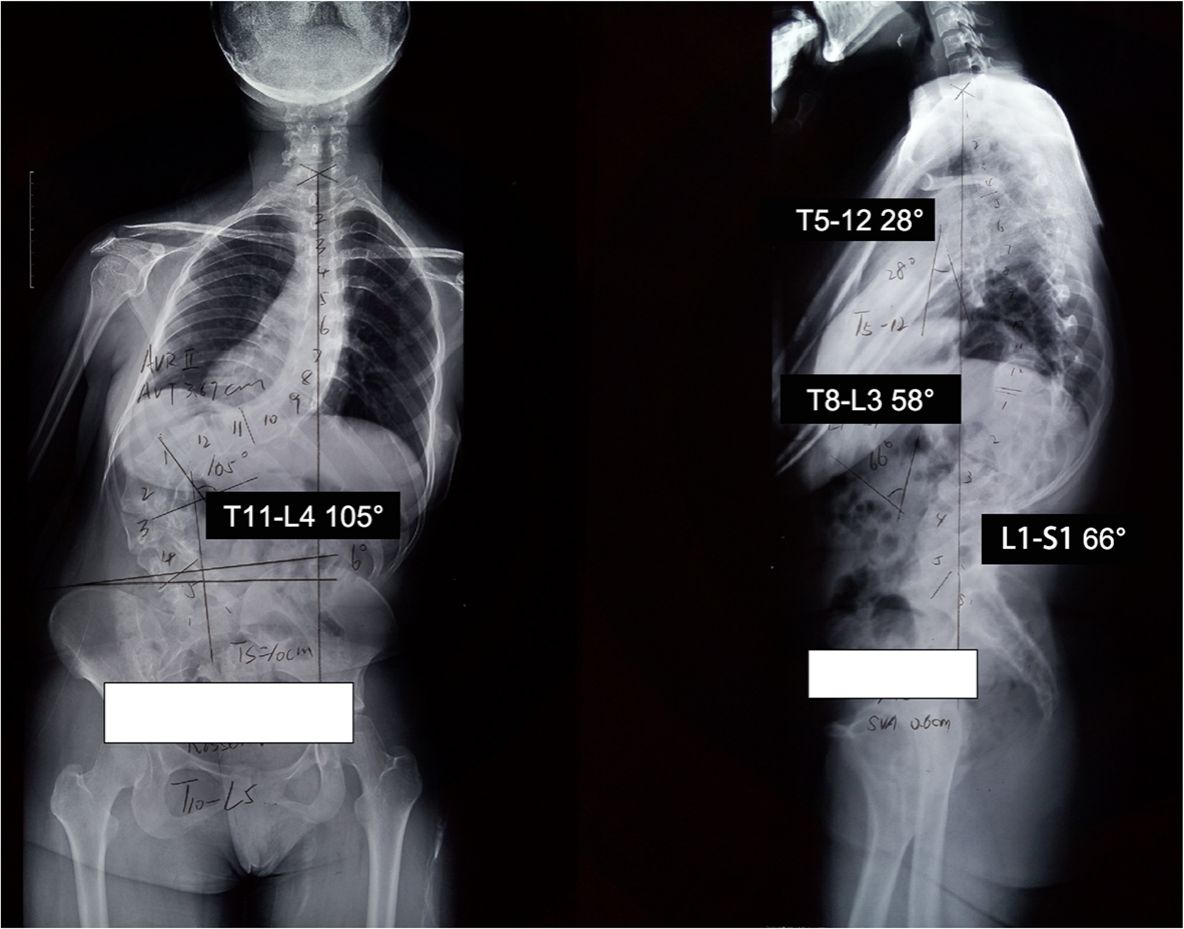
Standing anteroposterior and lateral radiographs
